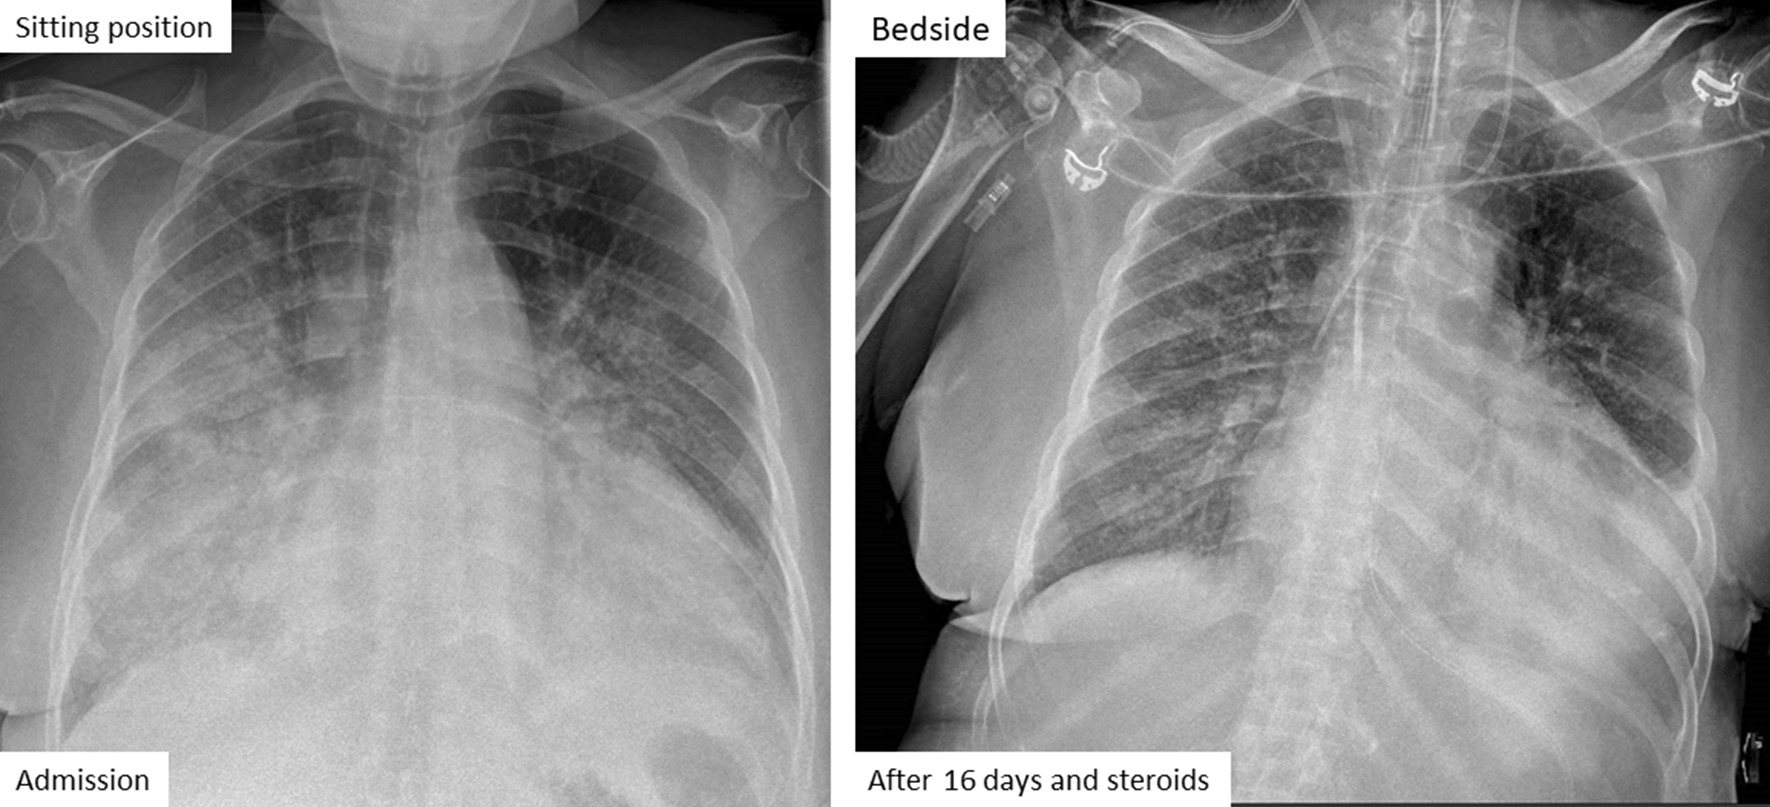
Chest X-ray examination on admission (left) and 16 days after the start of corticosteroids (right)
